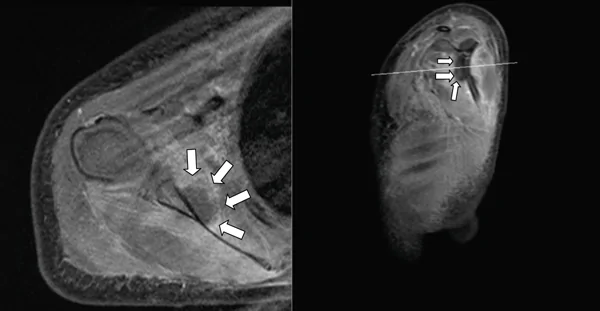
T1 fat saturation postcontrast MRI of the right shoulder with contrast demonstrating a midsubstance subscapularis abscess along the ventral border of the scapula measuring 2.6 by 1.6 by 0.8 cm.
